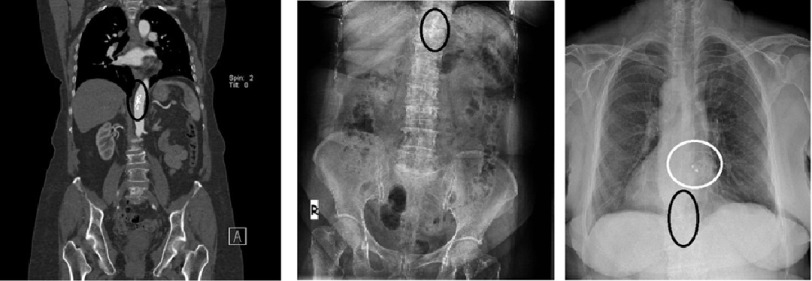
Embolized occluder device is marked in a black ring. Computed tomographic angiography (A) - also can be detected on X-ray before re-intervention (B). New and old-embolized devices can be detected on X-ray (C).
